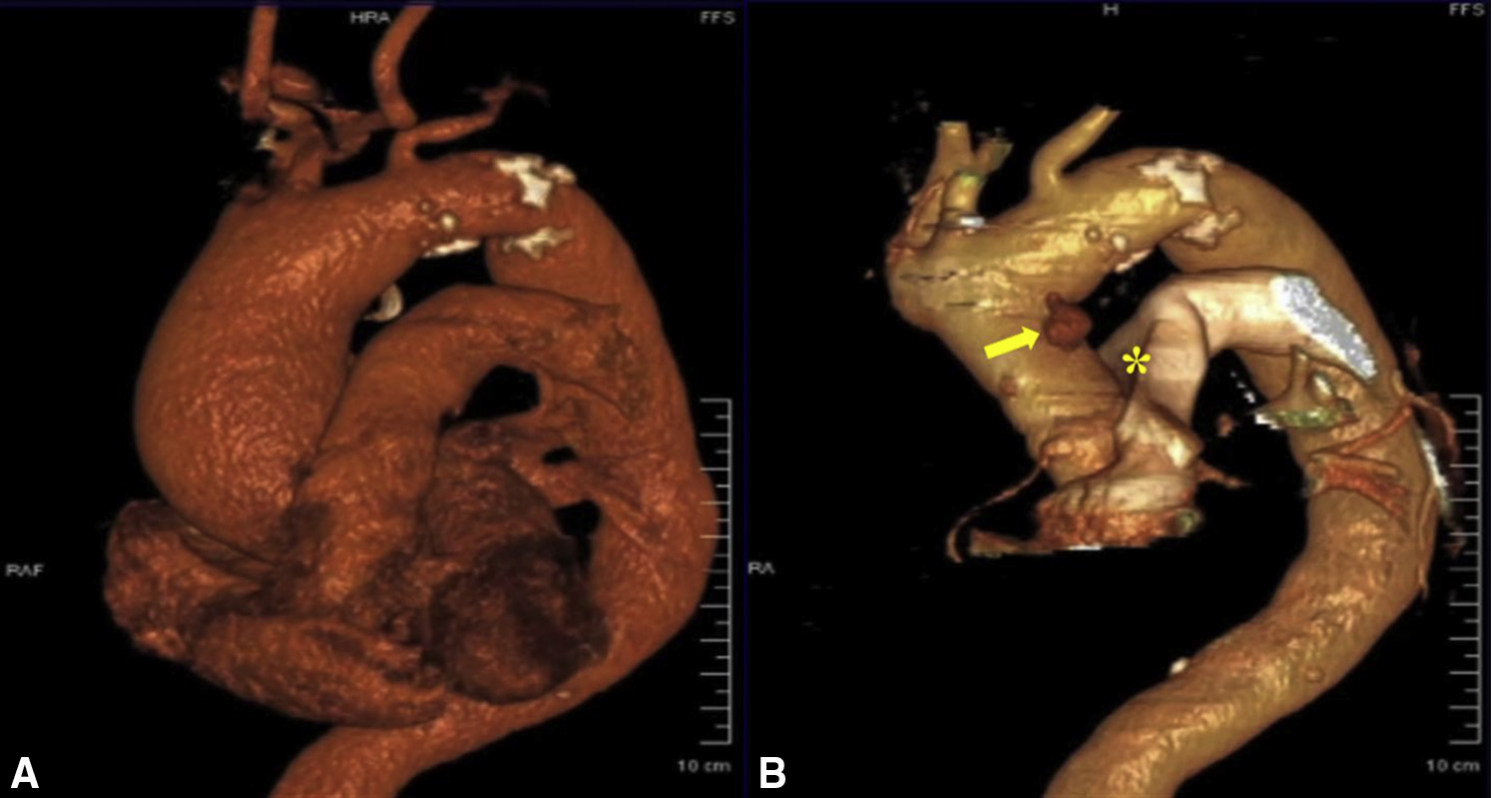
Ascending aortic aneurysm repair. A, On admission, preoperative cardiac computed tomography angiography demonstrated a 5.1-cm thoracic ascending aortic aneurysm. B, One month postoperatively, imaging showed a distal anastomotic leak with pseudoaneurysm formation (*arrow*) compressing the left main pulmonary artery (*asterisk*).
